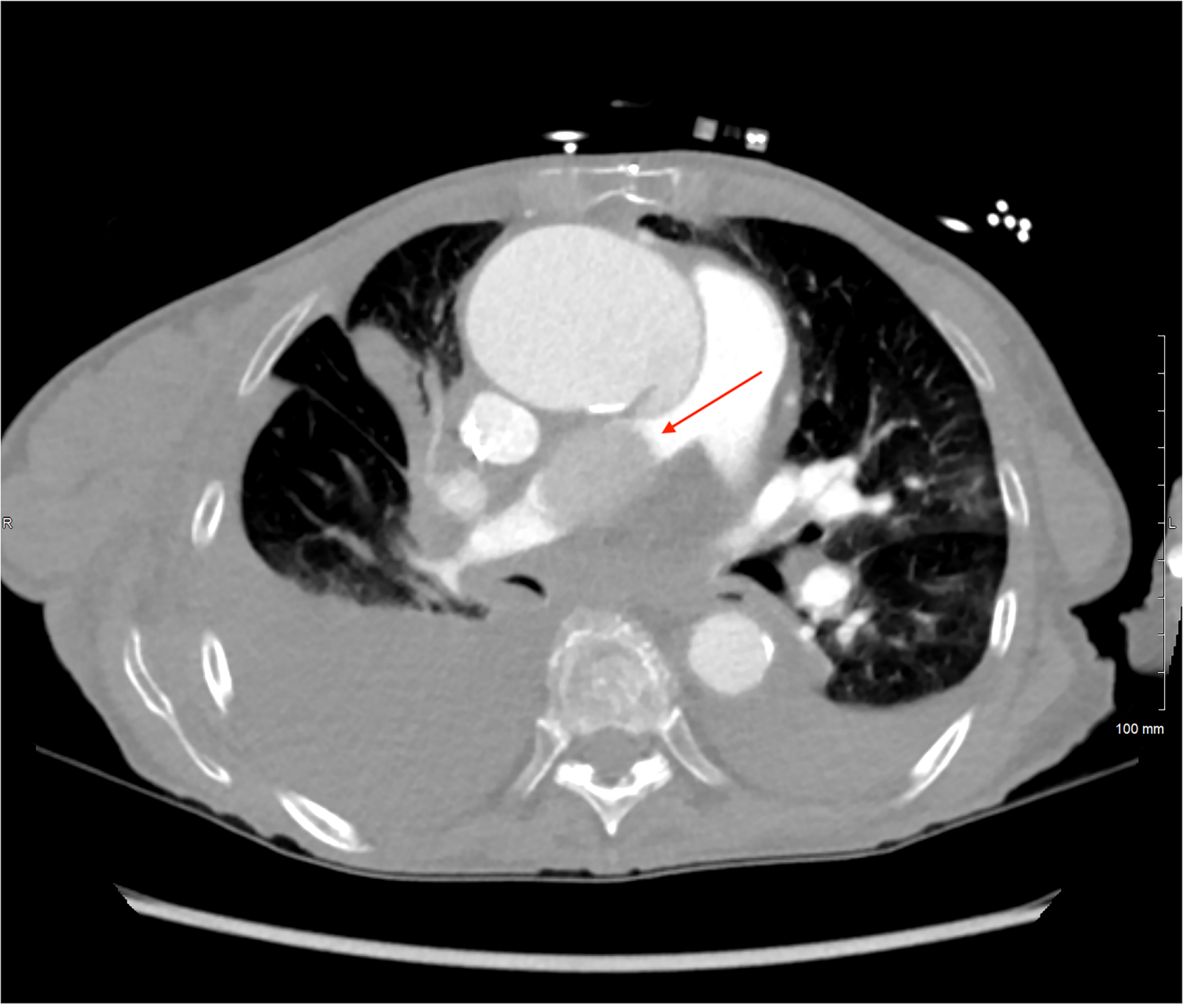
CT angiography of the thorax with pulmonary embolism protocol. This image depicts compression of the right pulmonary artery by the focal mass in the mediastinum measuring 3.1 × 4.3 cm (red arrow) which represents the mediastinal pseudoaneurysm. There is suboptimal filling of the right pulmonary arteries due to the compression. No discrete intraluminal filling defect is seen to suggest pulmonary artery embolism. The cardiac size was enlarged with no pericardial effusion, and reflux of contrast into the hepatic veins was noted, consistent with right heart dysfunction. [Color figure can be viewed at wileyonlinelibrary.com]
